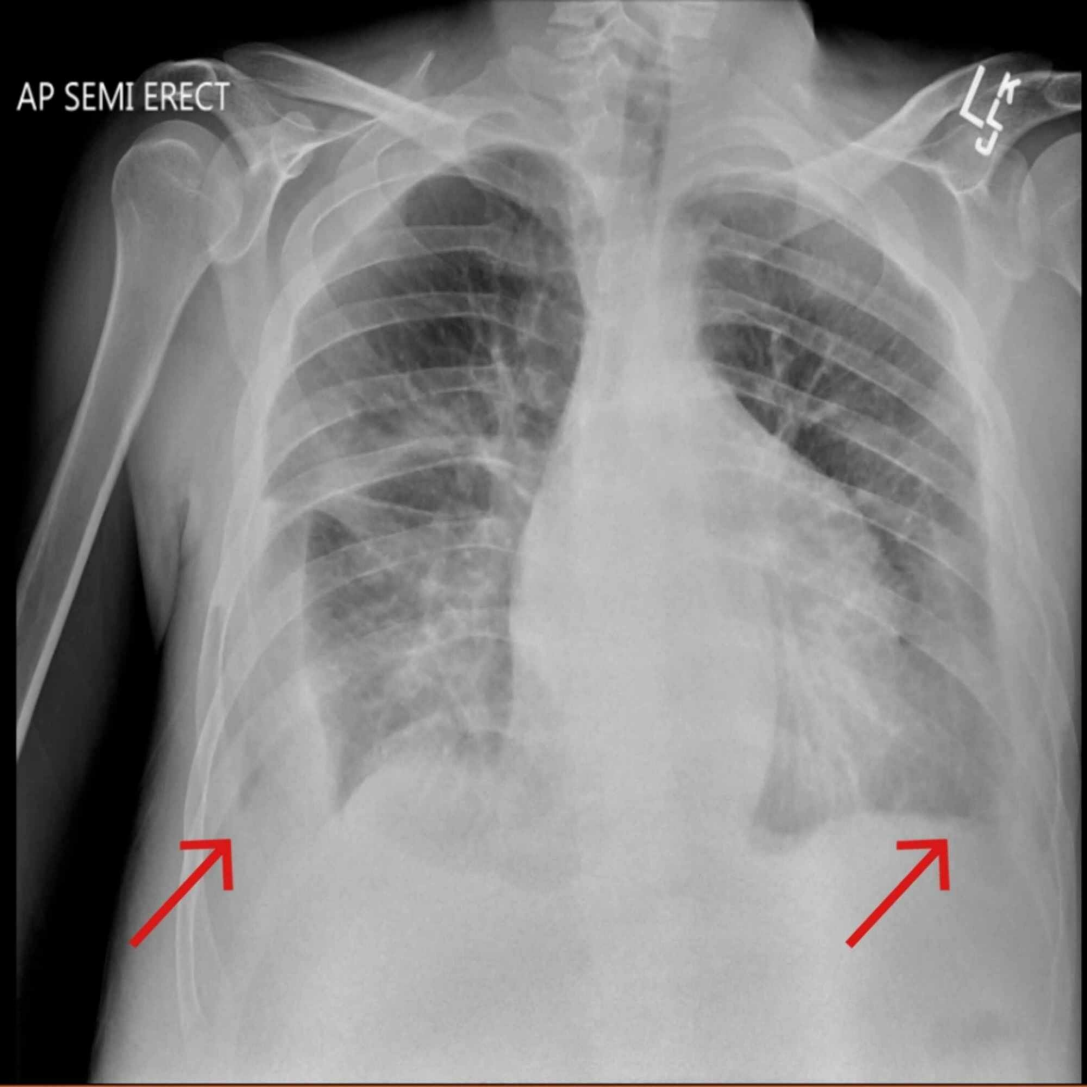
Initial chest X-ray - the arrows are showing the pleural effusions
